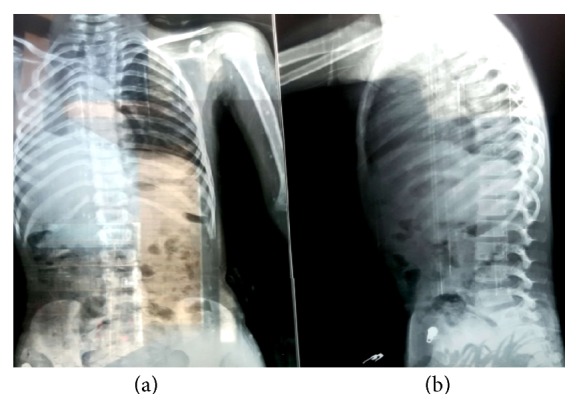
(a) X-ray showing dextrocardia, crowding of right-sided ribs, and tracheal shift to right side; (b) showing normal vertebrae.
